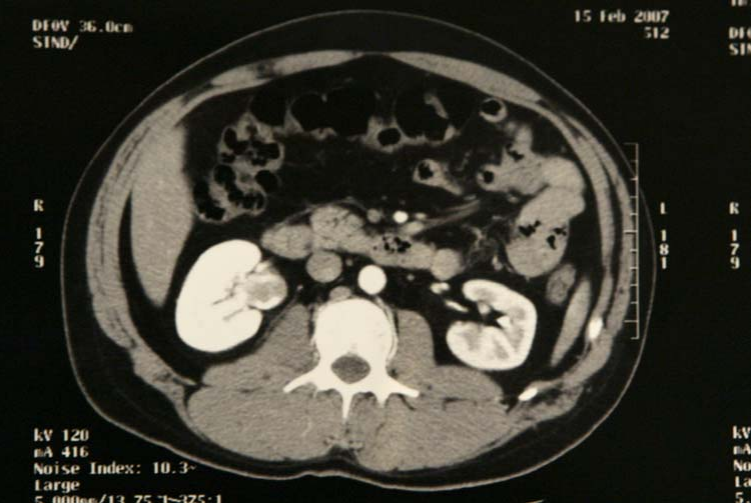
Axial computed tomography scan after intravenous administration of iodinated contrast material, arterial phase. A filling defect of soft tissue density is demonstrated within the right renal pelvis, which is also dilated. Differential diagnosis includes blood clot and tumor.
